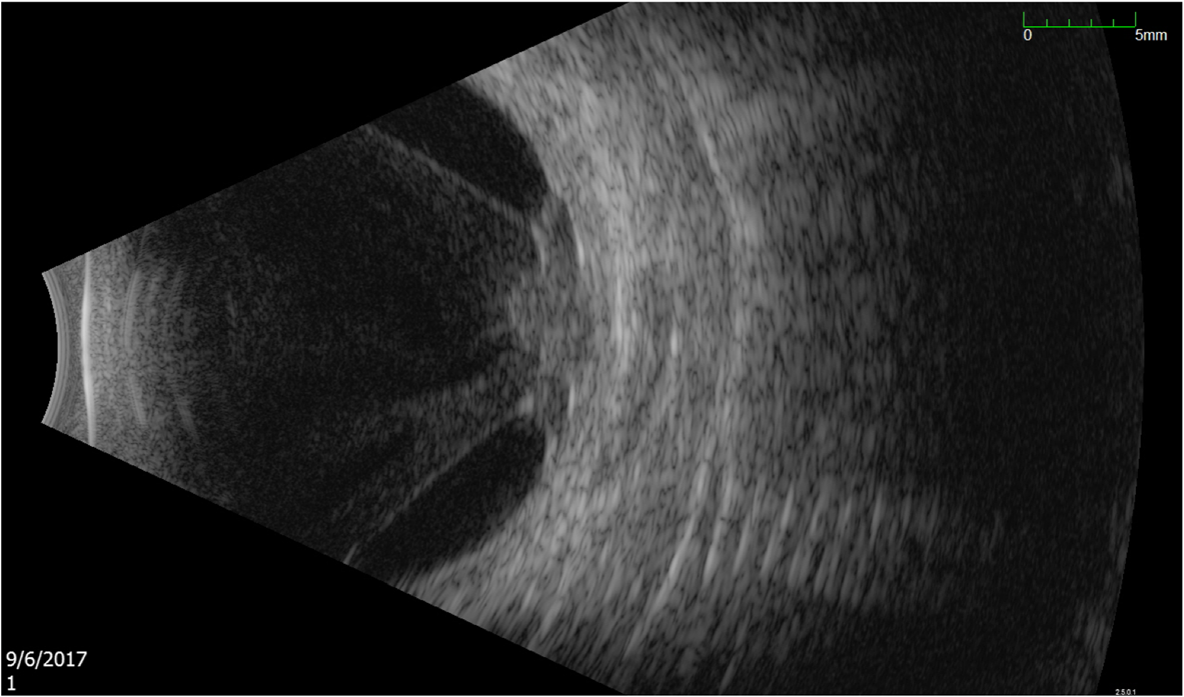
B-scan ultrasound demonstrating worsening nasal retinal traction at follow-up visit
